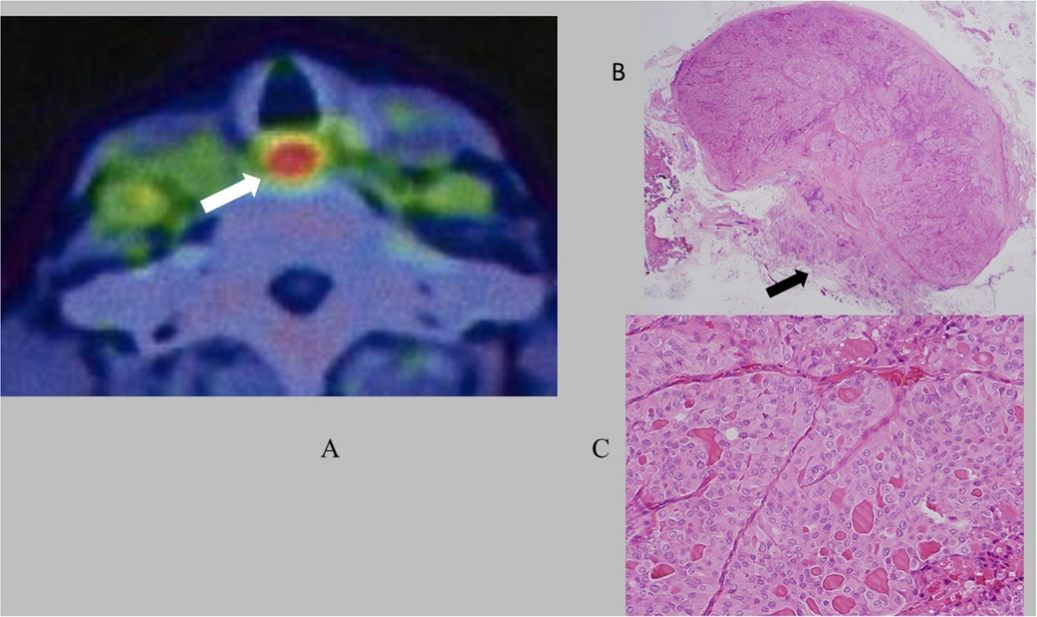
PET/CT and pathological findings. (A) Axial view of PET/CT study shows the recurrent tumor mass between the trachea and esophagus with high uptake of FDG at a peak standardized uptake value of 15.45. (B) Low-power magnification; arrow shows extracapsular extension. (C) High-power magnification. Pathological findings demonstrate poorly differentiated thyroid carcinoma.
